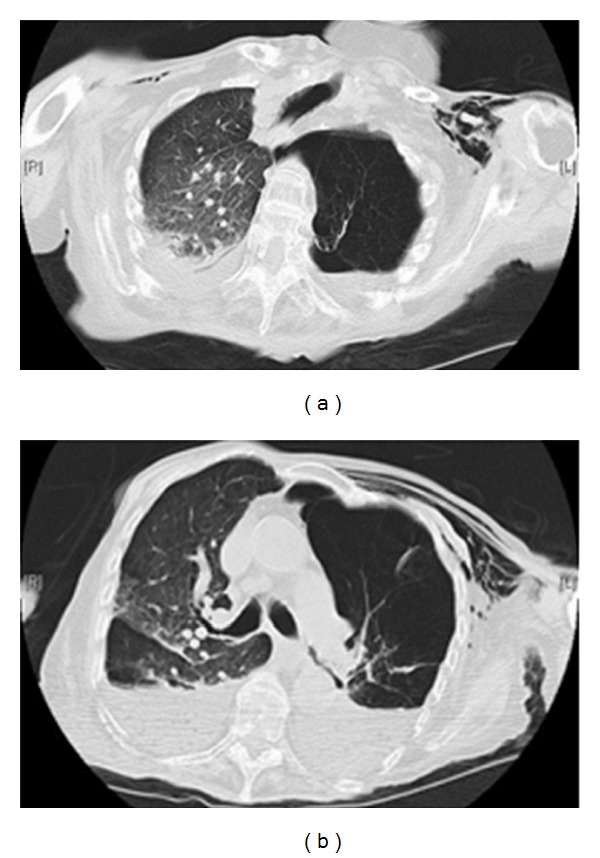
Axial CT scans on the patient in Figures 24 and 25 show that the chest drain was placed in bullous emphysema.
